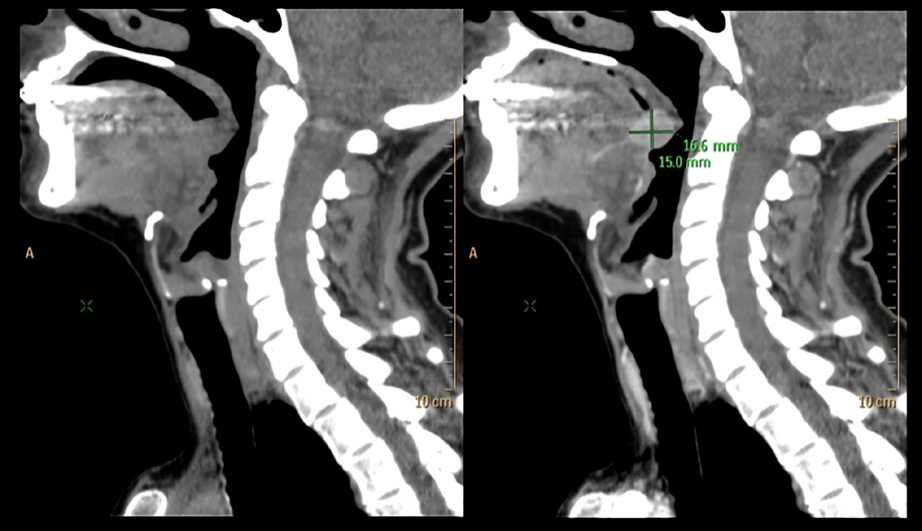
Head and Neck CT scan showing a nodule in the posterior aspect of the tongue (left) with contrast enhancement (right)
